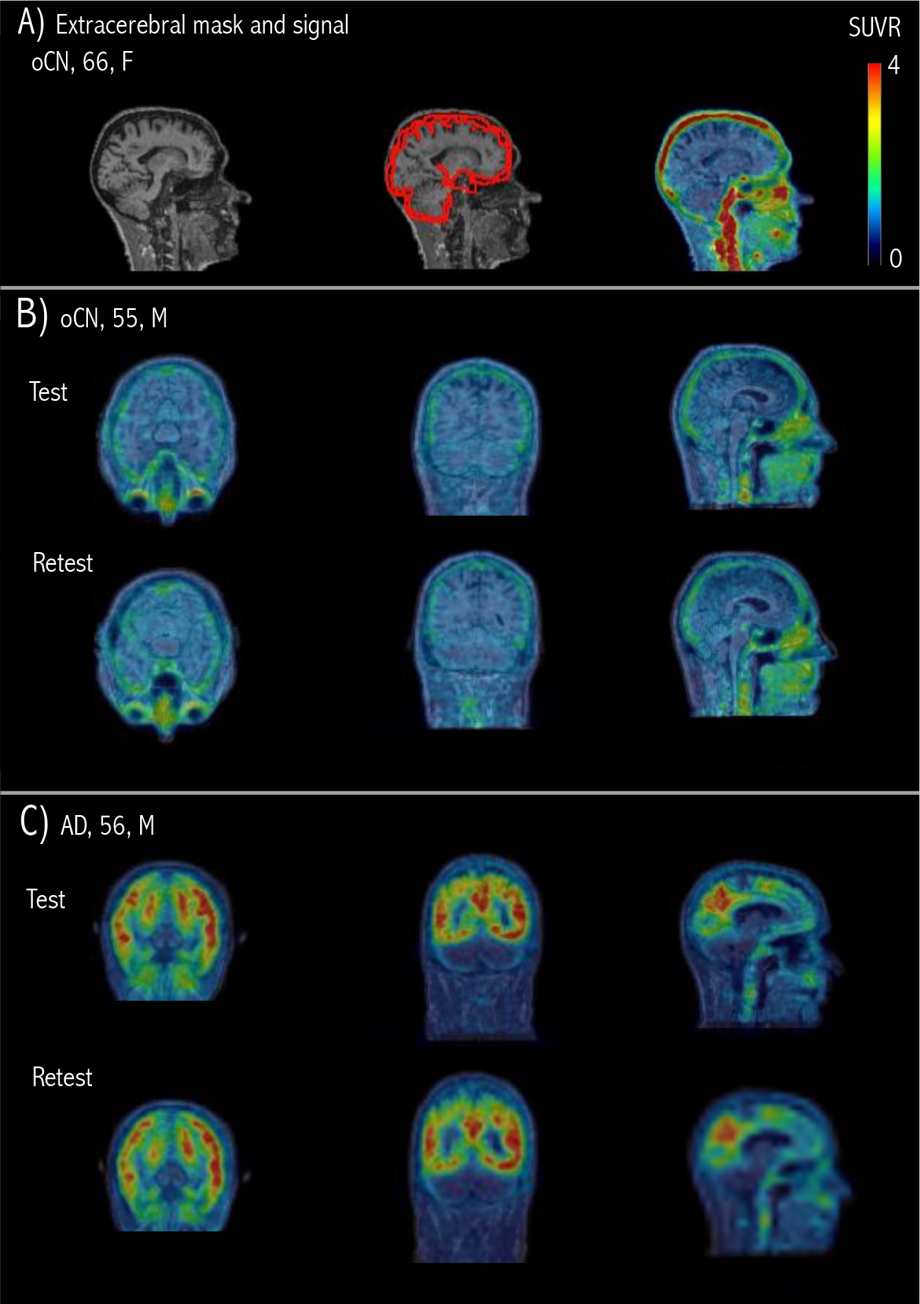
[^18^F]MK-6240 SUVR_90–110_PET images (usingCerGM as reference) overlaid on simultaneously acquired structural MRI.(A) Example oCN subject with high extracerebral signal and usedextracerebral mask (red). The mask was created by dilating theFreeSurfer-generated cortical segmentation by 2–5 mm outwardperpendicularly to the cortical surface. (B) Example oCN subject TestPET (top) and Retest PET (bottom), showing low cerebral uptake and amild but noticeable uptake in extracerebral regions, including themeninges. (C) Example AD subject Test PET (top) and Retest PET (bottom),showing high uptake in numerous cortical areas and noticeable uptake inextracerebral regions, including the meninges.
